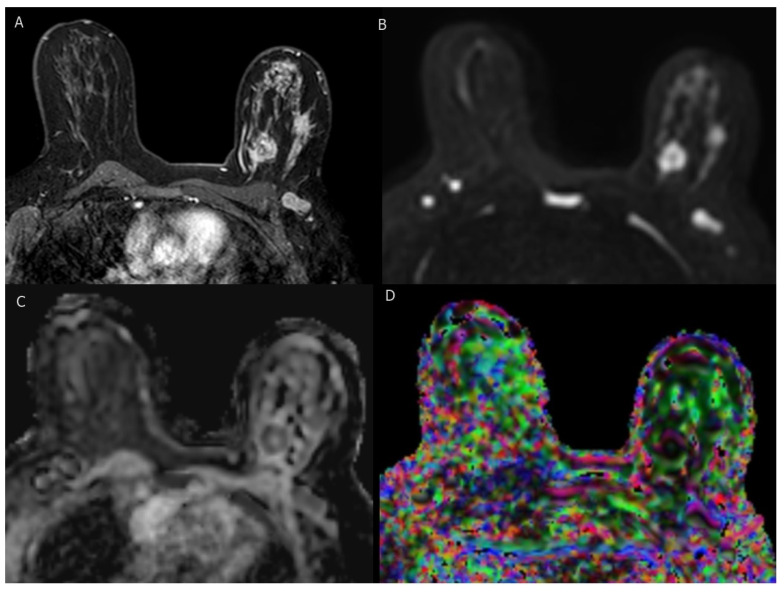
BI-RADS category 5: 37-year-old woman with triple-negative invasive ductal carcinoma. (A) axial T1-weighted fat-saturated dynamic contrast-enhanced MR image shows two irregular masses of heterogenous enhancement with ipsilateral suspicious axillary LN. (B–D) axial DWI (b = 1000), corresponding ADC map, and DTI image (colored FA map ) show marked diffusion restriction of two masses and axillary LN with low ADC values ($0.89\times 10^{-3}$ mm ${}^{2}$/s, $0.91\times 10^{-3}$ mm ${}^{2}$/s and $0.93\times 10^{-3}$ mm ${}^{2}$/s ) and high FA values (0.57, 0.56 and 0.54), respectively.
